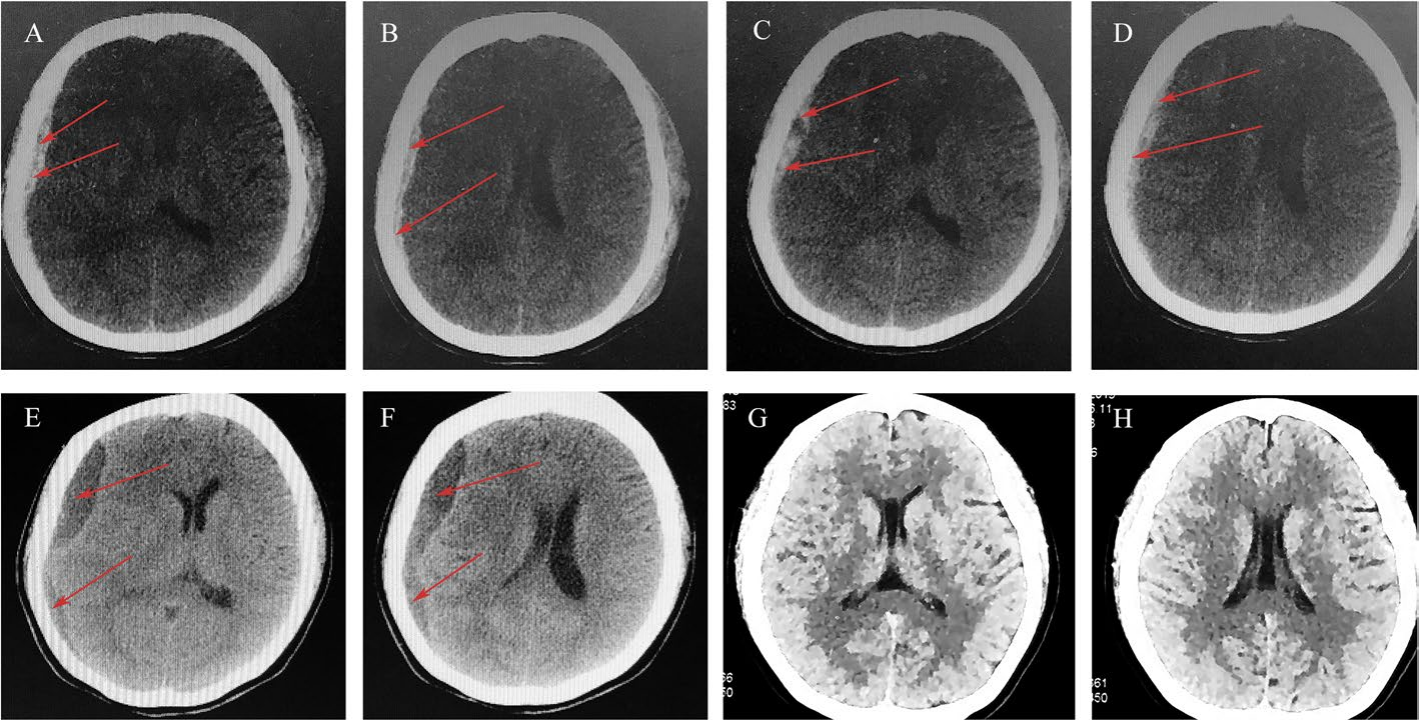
CT scan images for patient 5. Initial brain CT revealed right subdural hematoma (red arrows) (A, B). CT images obtained after starting atorvastatin plus low-dose dexamethasone therapy. C, D Day 5. E, F Day 14. G, H Six weeks
